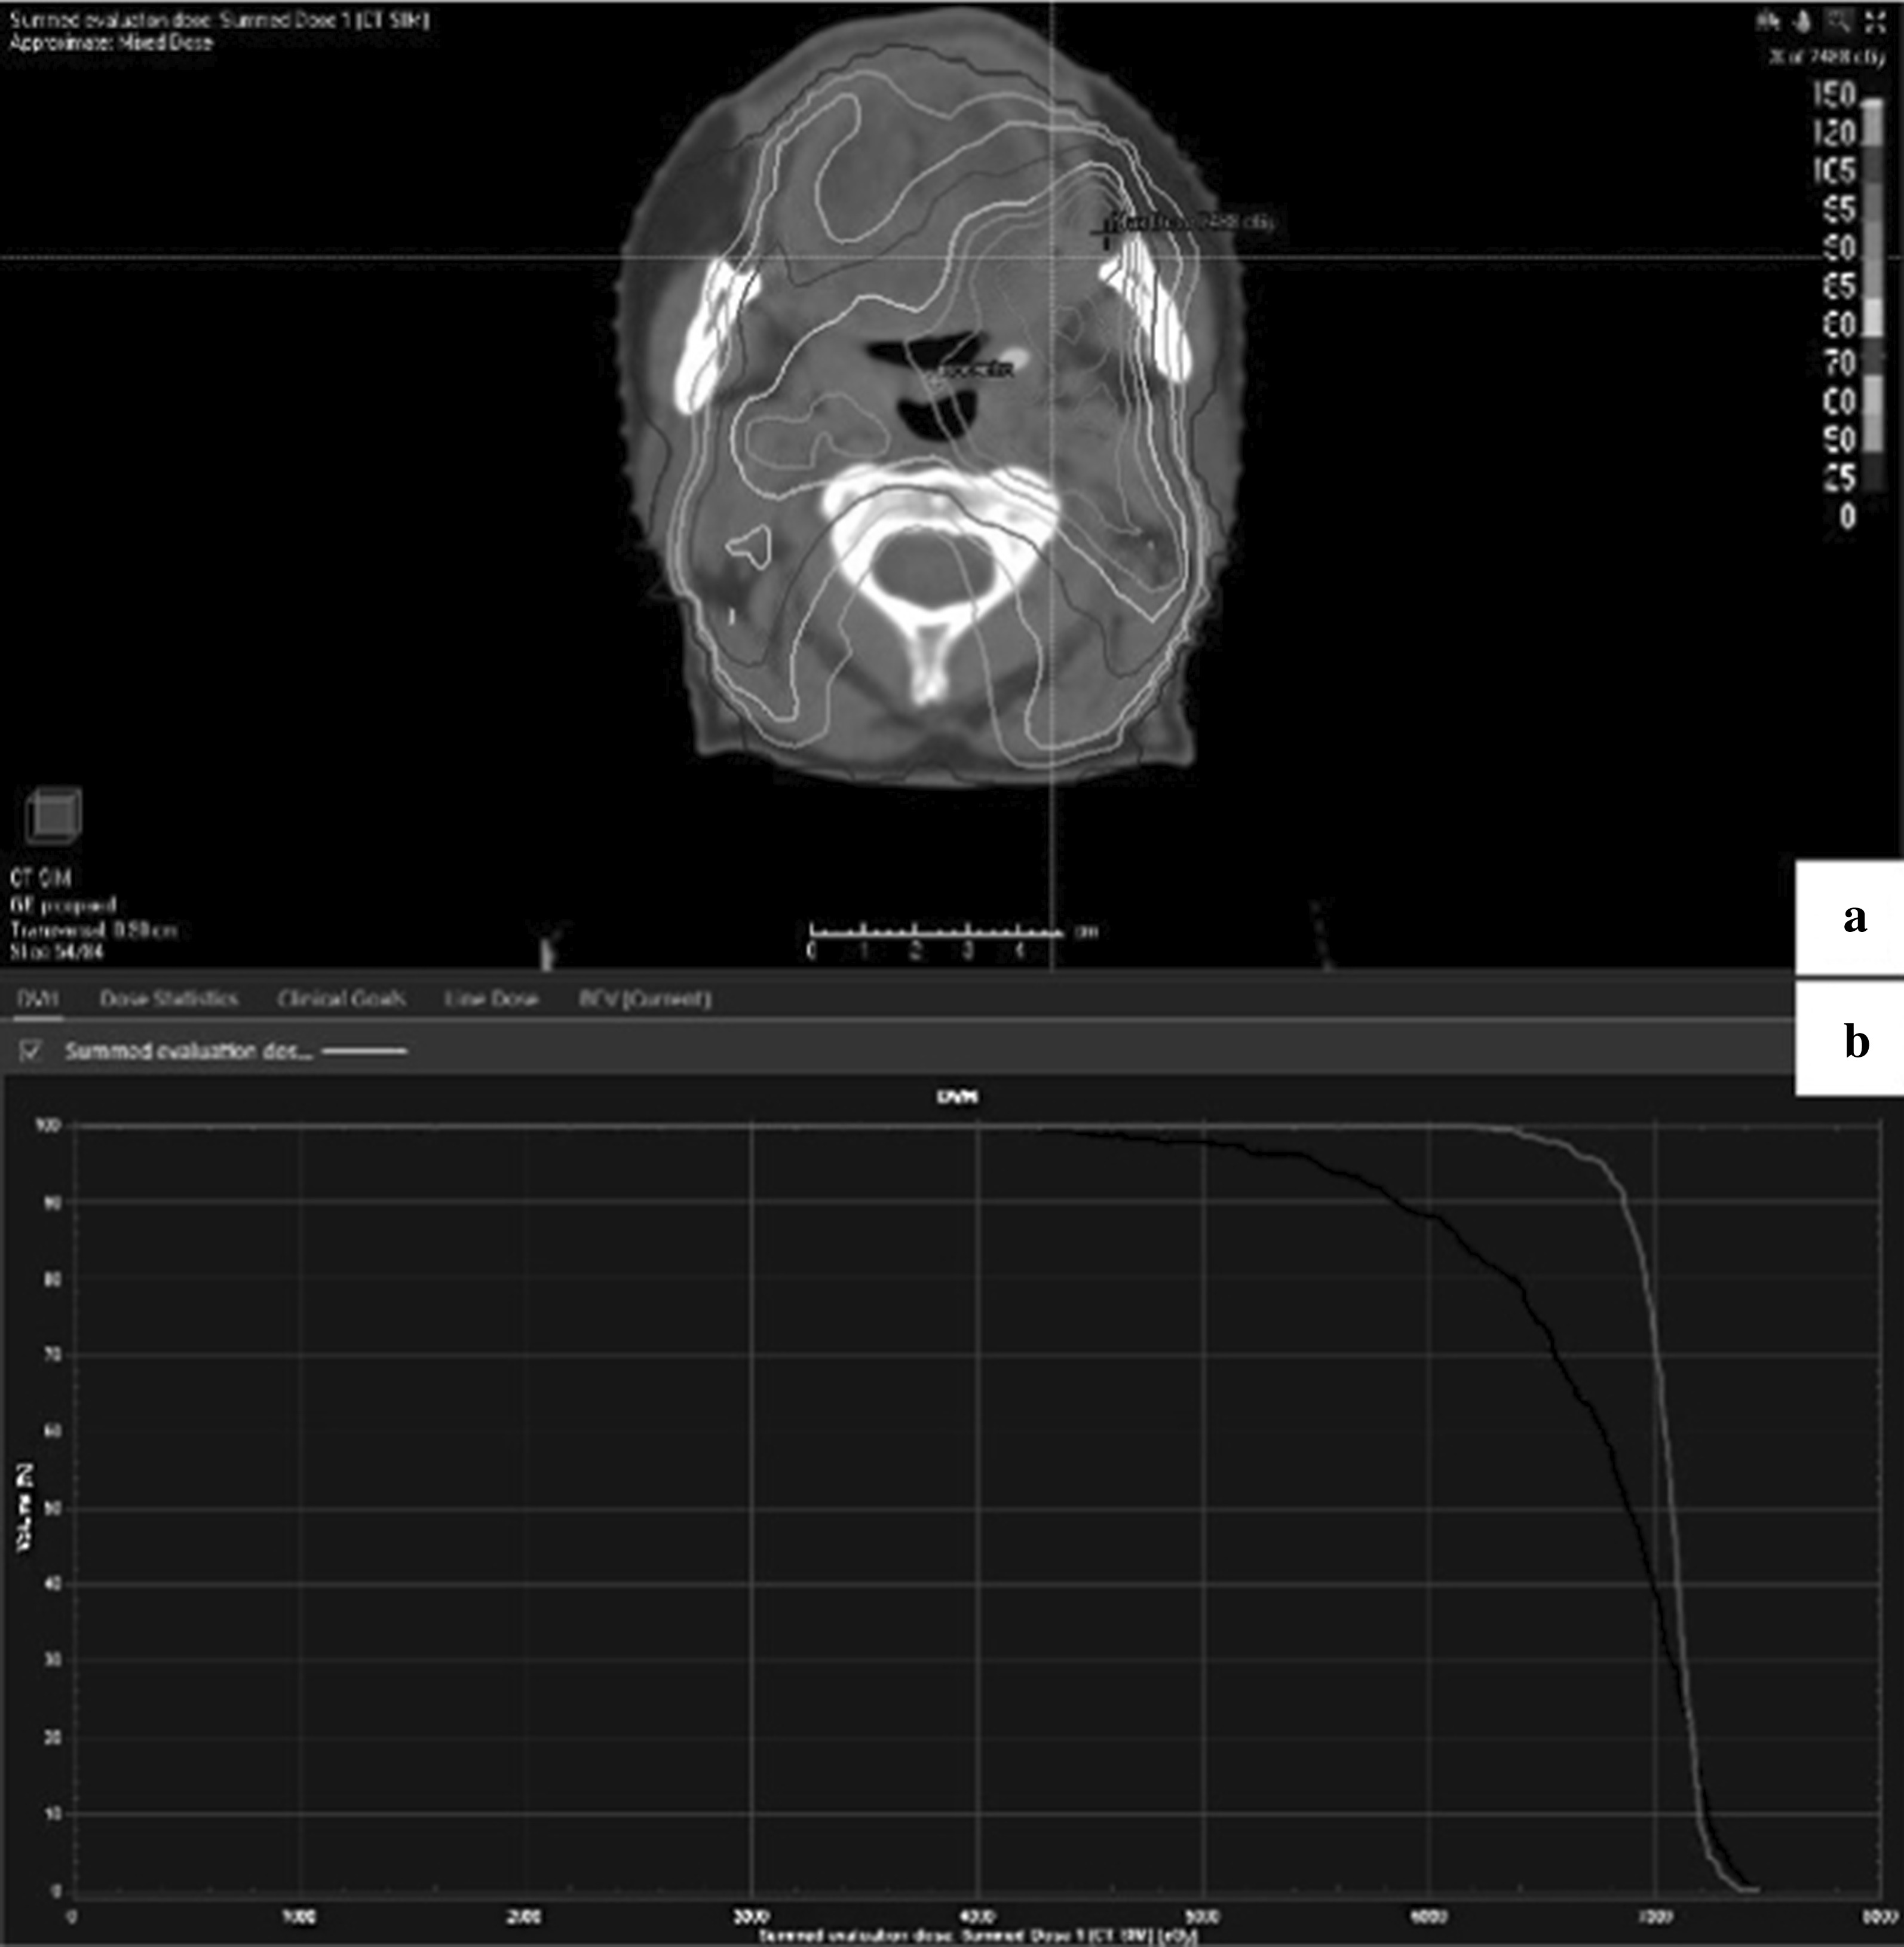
Example of oral cavity recurrence “out of field”. a Planning CT displayed in transverse plans with isodose lines. b Dose-volume histograms (DVH) showing an appropriate coverage of MTV_pre_. MTV_rec_ is outside dose coverage region (D99 = 44.7 Gy and MTV_rec_ D95 = 47.4 Gy). Light gray contour: MTV_pre_. Black contour: MTV_rec_
